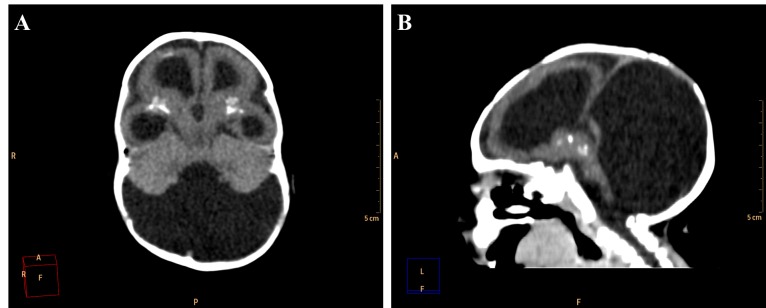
Figure 9. Axial (A) and sagittal (B) non-contrast brain CT show a 3 month old infant female evidencing hypoplastic cerebellar vermis, cystic dilatation of the fourth ventricle and enlargement of the posterior fossa, representing Dandy-Walker syndrome.
